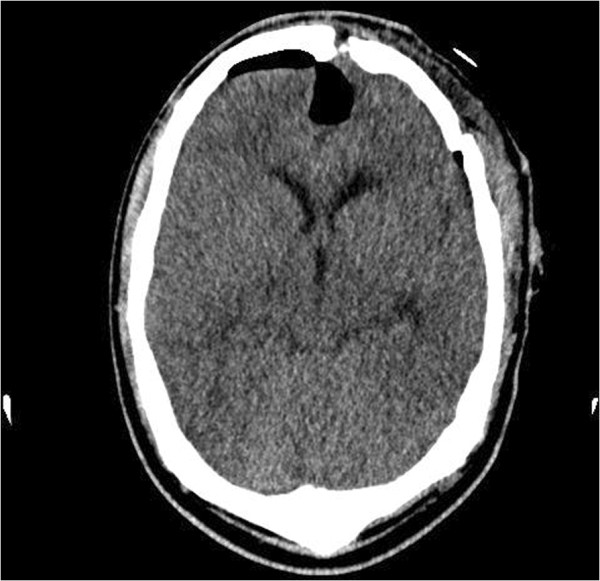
Post-operative computed tomography scan showing successful craniotomy.
